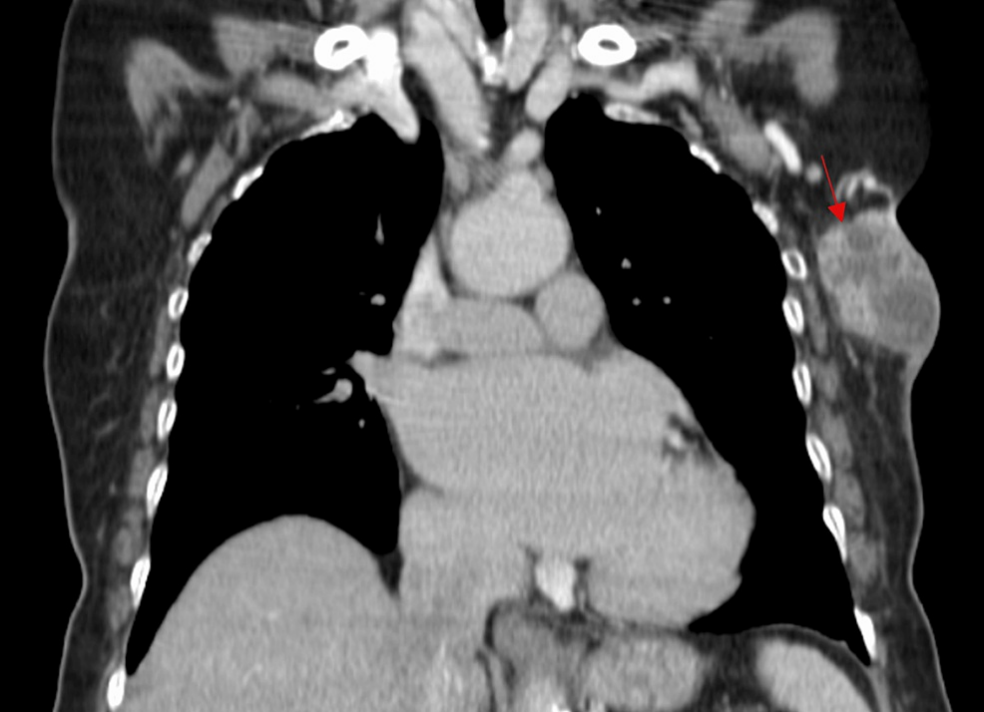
Coronal enhanced CT acquisition of the thorax revealing a lesion with heterogeneous contrast uptake, consisting of a solid and multicystic component, involving the upper outer quadrant of the left breast (red arrow). CT, computed tomography
